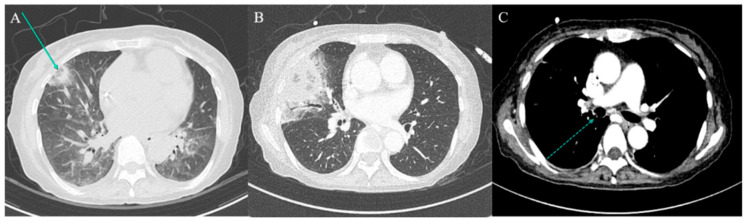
Serial chest computed tomography (CT) findings. (A) Low-dose chest CT performed on post-transplant day (PTD) 15 due to persistent haziness on chest radiograph reveals multiple nodular consolidations, including a reverse halo sign (arrow) in the right middle lobe (RML). (B,C) Contrast-enhanced chest CT on PTD 28 shows a new luminal nodular lesion in the right main bronchus (dotted arrow) and progression of the previously noted RML consolidation.
